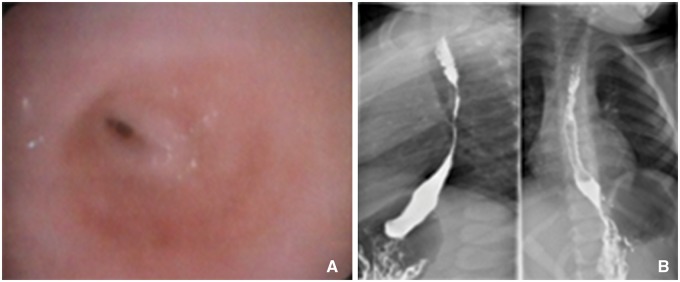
A 3-year-old female patient with an accidental caustic ingestion: (A) shows an endoscopic picture of esophageal stenosis, and (B) shows an x-ray image of esophageal stenosis.
